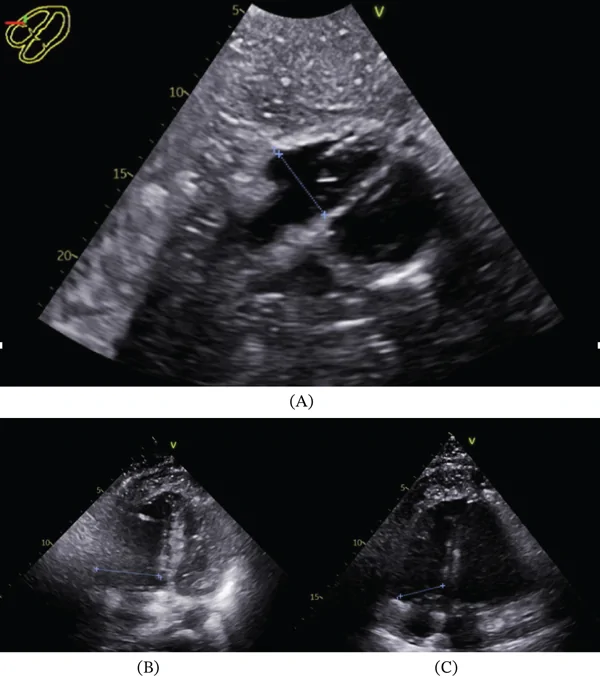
(A) Initial subcostal echocardiogram demonstrating a moderately dilated RV with severely reduced systolic function. (B) Apical four‐chamber view showing an RV base diameter of 4.29 cm (normal: 3.5–4 cm) and tricuspid annular plane systolic excursion (TAPSE) of 11.1 mm (abnormal < 16 mm). (C) Repeat apical view 3 days posttreatment with RV base reduced to 3.08 cm and TAPSE improved to 19.82 mm, consistent with recovery of RV function.
